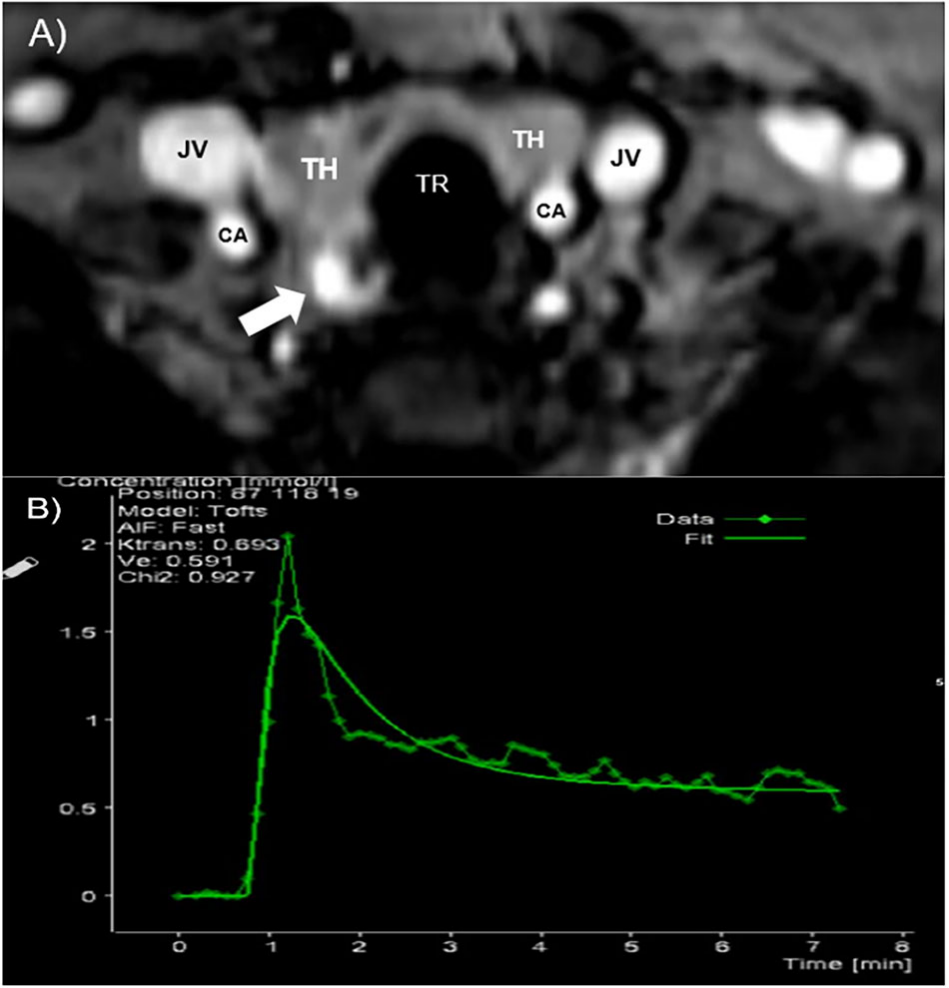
MR image of a 32-year-old man with PHPT. A Axial T1 TWIST sequence demonstrates a hyperintense lesion in the right inferior quadrant (JV jugular vein, CA carotis artery, TH thyroid lobes, TR trachea). B Dynamic time-contrast concentration curve of a parathyroid adenoma
